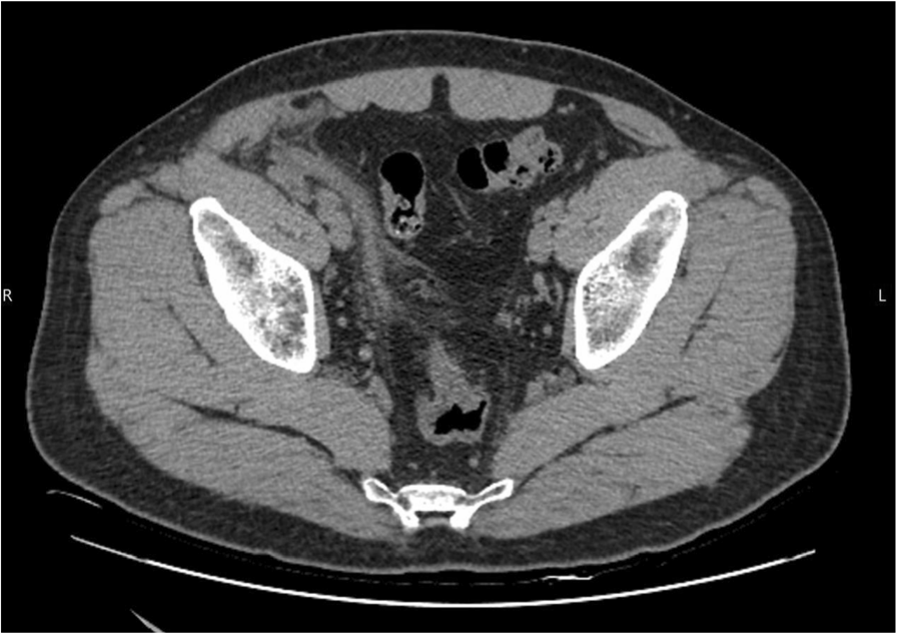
Non-enhanced axial CT image revealed dilated right vas deferens with peripheral fat stranding (white arrows)
